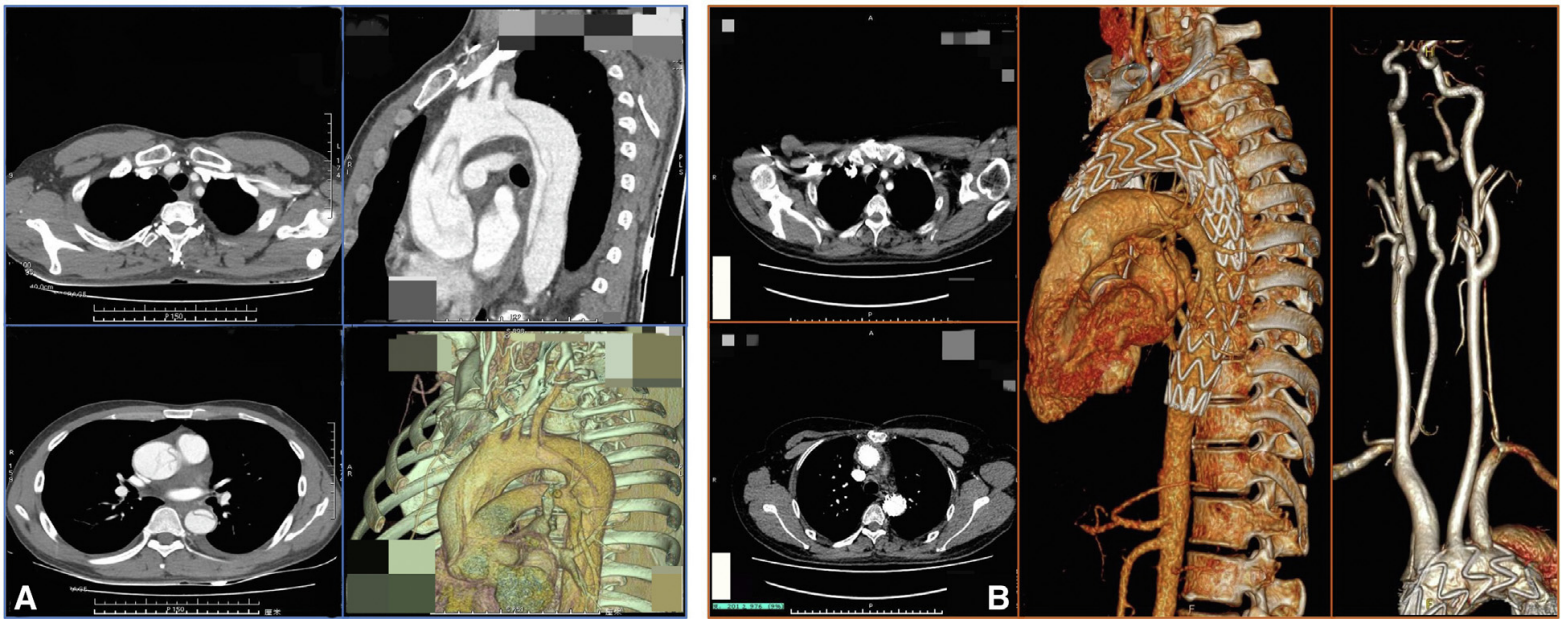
Images of computed tomography angiography (*CTA*). A, Preoperative CTA. B, Postoperative CTA.
